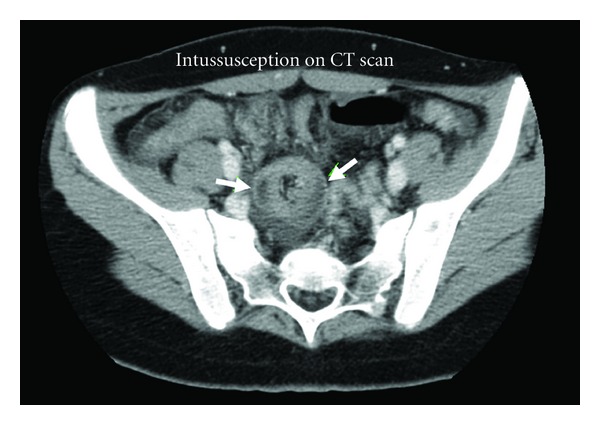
CT scan of abdomen showing intussuscepted bowel.
